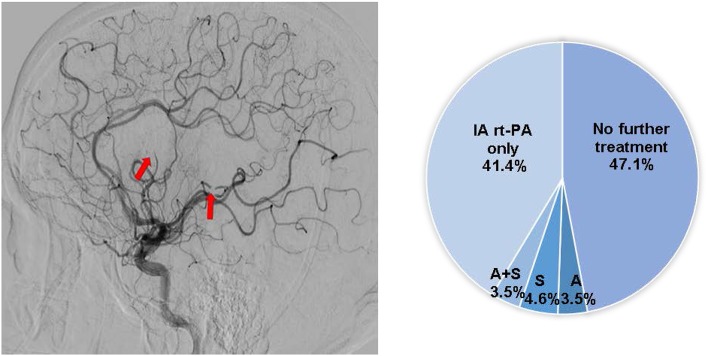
Case presentation #2. A 65-year-old with right sided weakness and aphasia, NIHSS 18, last known well 8 h prior to presentation, ASPECTS 9, with a left M1 occlusion on head CTA. Post left MCA MT, distal embolization occurred into multiple M3 branches. Pie chart depicts the respondents' treatment preferences. A, aspiration only; S, stent-retriever only; A+S, aspiration and stent-retriever.
